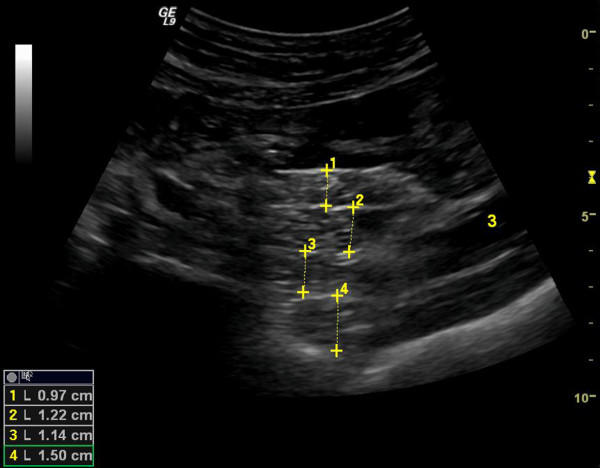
Mesenteric fat thickness measurement/ detailed legend: mesenteric fat thickness estimated by ultrasonography.  The mesenteric leaves are indicated by crosses.
